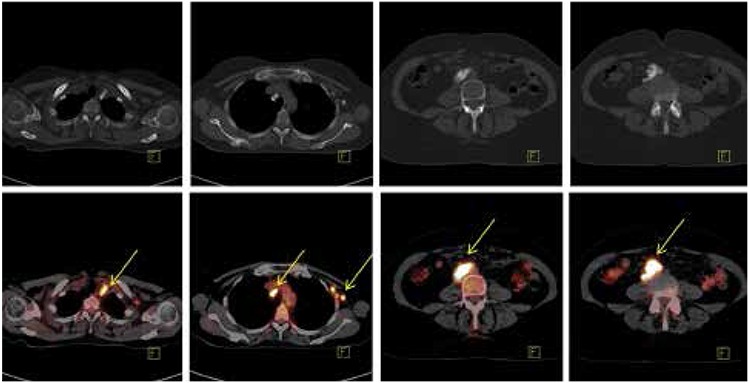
Calcified lymph nodes in the mediastinum and abdomen demonstrate high fludeoxyglucose uptake (arrows). Average SUVmax=8.78, highest SUVmax=12.7. Upper row: Calcifications as displayed by computed tomography (bone window). Lower row: Corresponding positron emission tomography/computed tomography images (soft tissue window)
